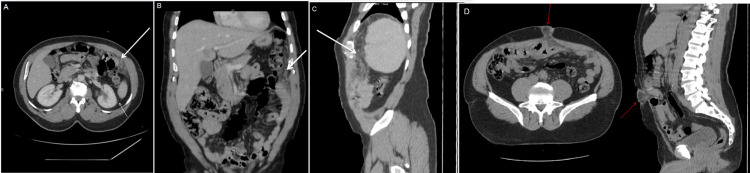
CT scan of the abdomen after IV contrast administration (A) axial, (B) coronal, and (C) sagittal reformatted images demonstrate the greater omentum is infiltrated by linear and nodules of soft tissue at the left upper abdomen (misty omentum), white arrows. Note: Clear appearance of normal intra-abdominal fat (thin white arrow). (D) There is fat-containing umbilical hernia with fat stranding. The hernial neck measures about 1.5 cm, and the hernial sac measures about 3.6 x 2.3 cm CT: computed tomography, IV: intravenous
